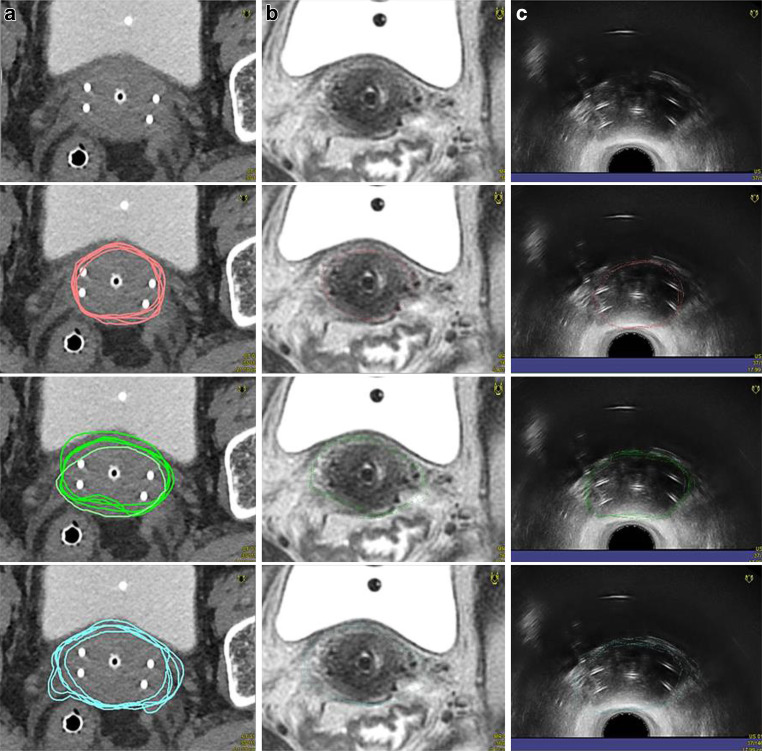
Example contours of a patient with FIGO IIB cervical carcinoma at time of brachytherapy with the applicator in place on computed tomography (CT) (a), magnetic resonance imaging (MRI) (b), and transrectal ultrasound (TRUS) (c). Contours from all observers are projected on the CT dataset: MRI contours in *pink*, TRUS contours in *green*, CT contours in *blue*
